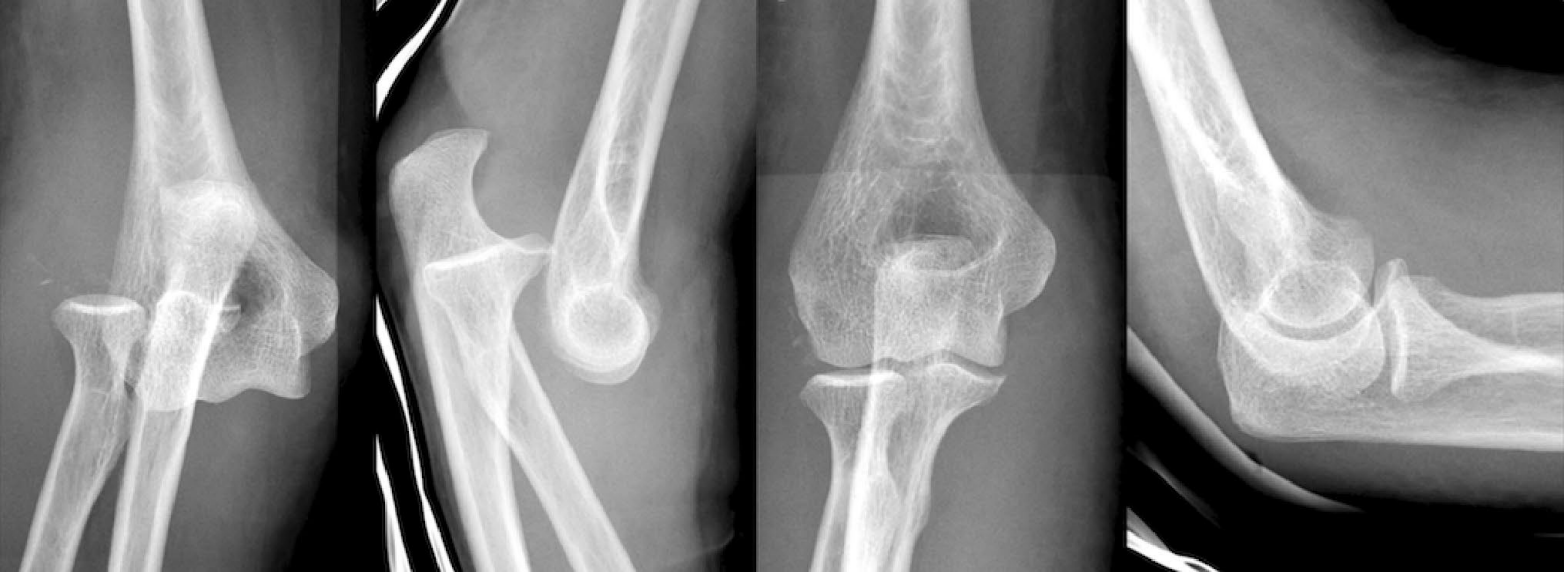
Antero-posterior and lateral radiographs of simple elbow dislocation. a, b Posterior elbow dislocation. c, d After closed reduction
